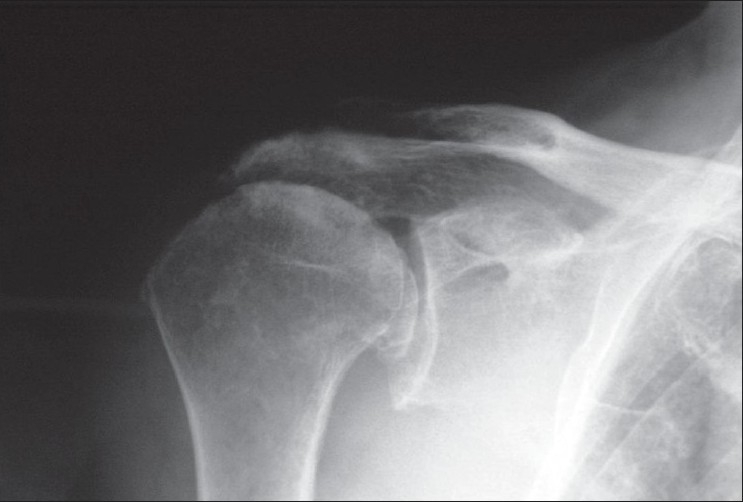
Preoperative X-ray showing osteoarthritis of the shoulder
